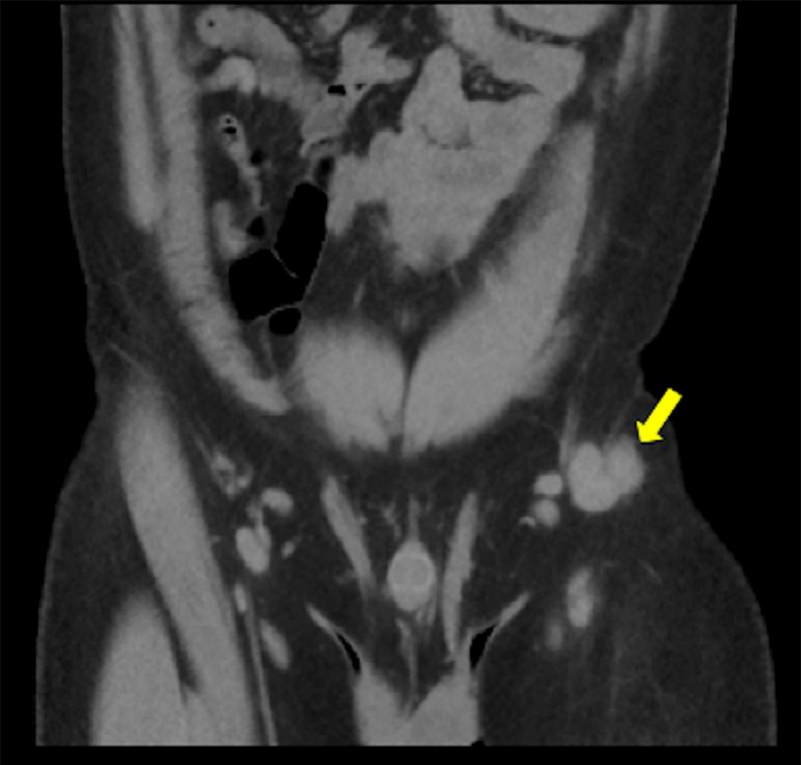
Coronal contrast enhanced CT of the pelvis demonstrating enlarged left inguinal lymph nodes (arrow).
